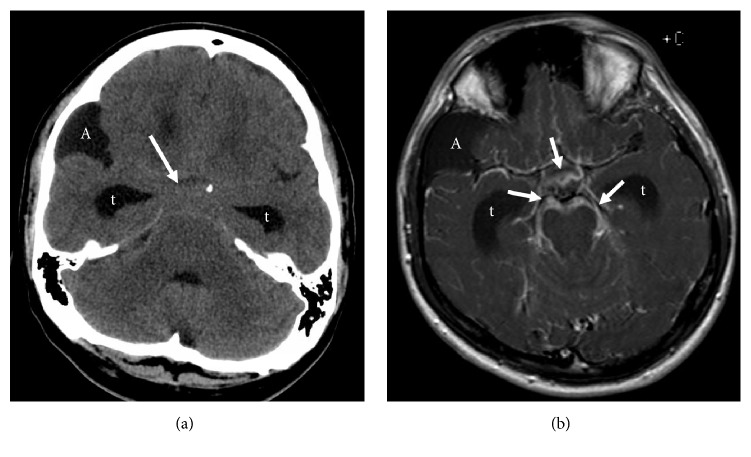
Axial noncontrast CT Head (a) and postcontrast T1 (b) MR images at the time of admission. Isodense basal leptomeningitis (arrow) on CT (a) with corresponding thick enhancement (arrows) on MRI (b). Hydrocephalus with dilated temporal horns (t). Incidental arachnoid cyst (A).
